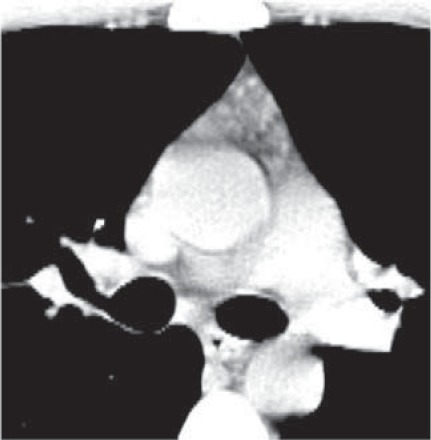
29-y/o female with myasthenia gravis; CT scan shows remaining thymic mass with early fatty infiltration, pathologic diagnosis - thymic hyperplasia
